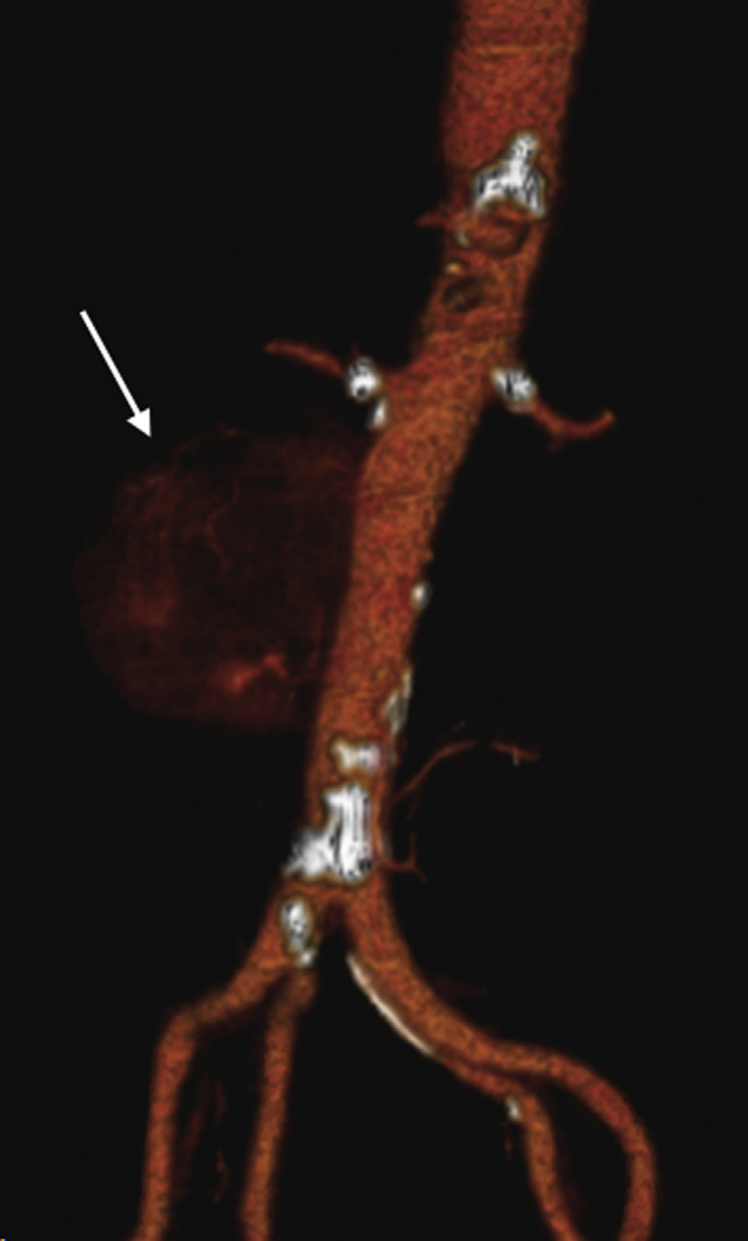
Three-dimensional reconstruction of computed tomography angiogram demonstrating infrarenal aortic pseudoaneurysm with a focus of active bleeding (*arrow*).
